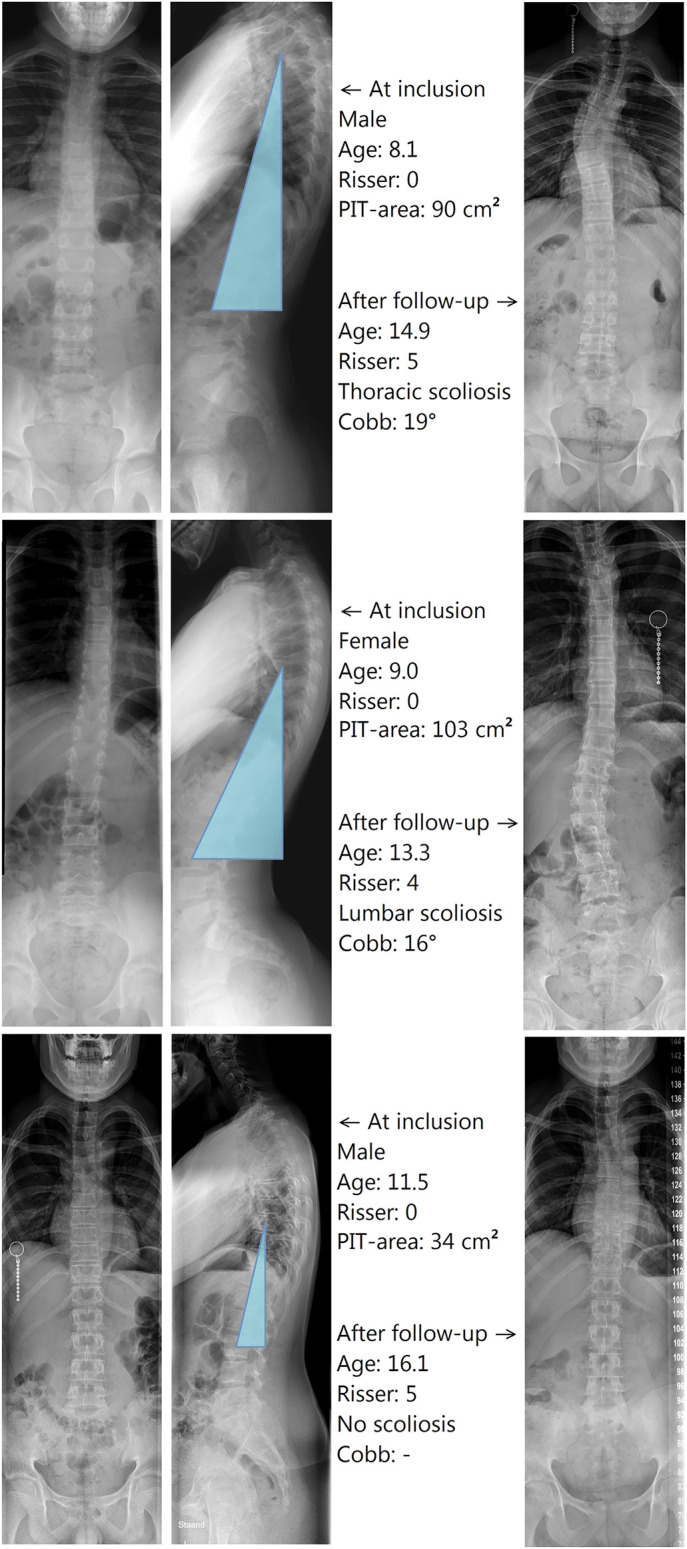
Radiographs of 3 study participants, demonstrating a larger PIT area before the development of scoliosis compared with the PIT area of patients who did not develop scoliosis. Note the tall and slender PIT in the patient with thoracic scoliosis compared with the wider and lower PIT in the patient with (thoraco)lumbar scoliosis.
